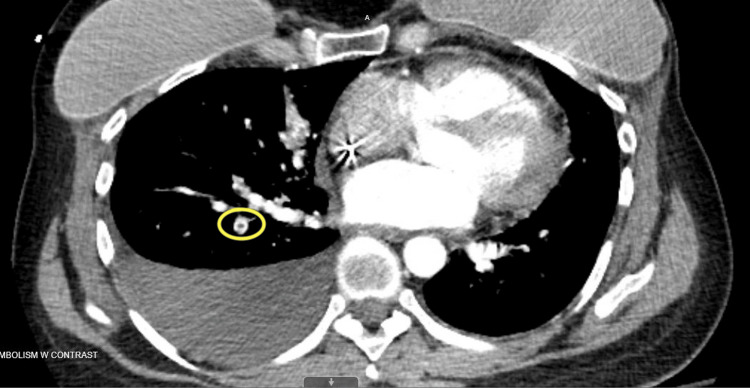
CT pulmonary embolism protocol indicating right lower lobe segmental branch pulmonary artery embolism (indicated by the yellow circle)
